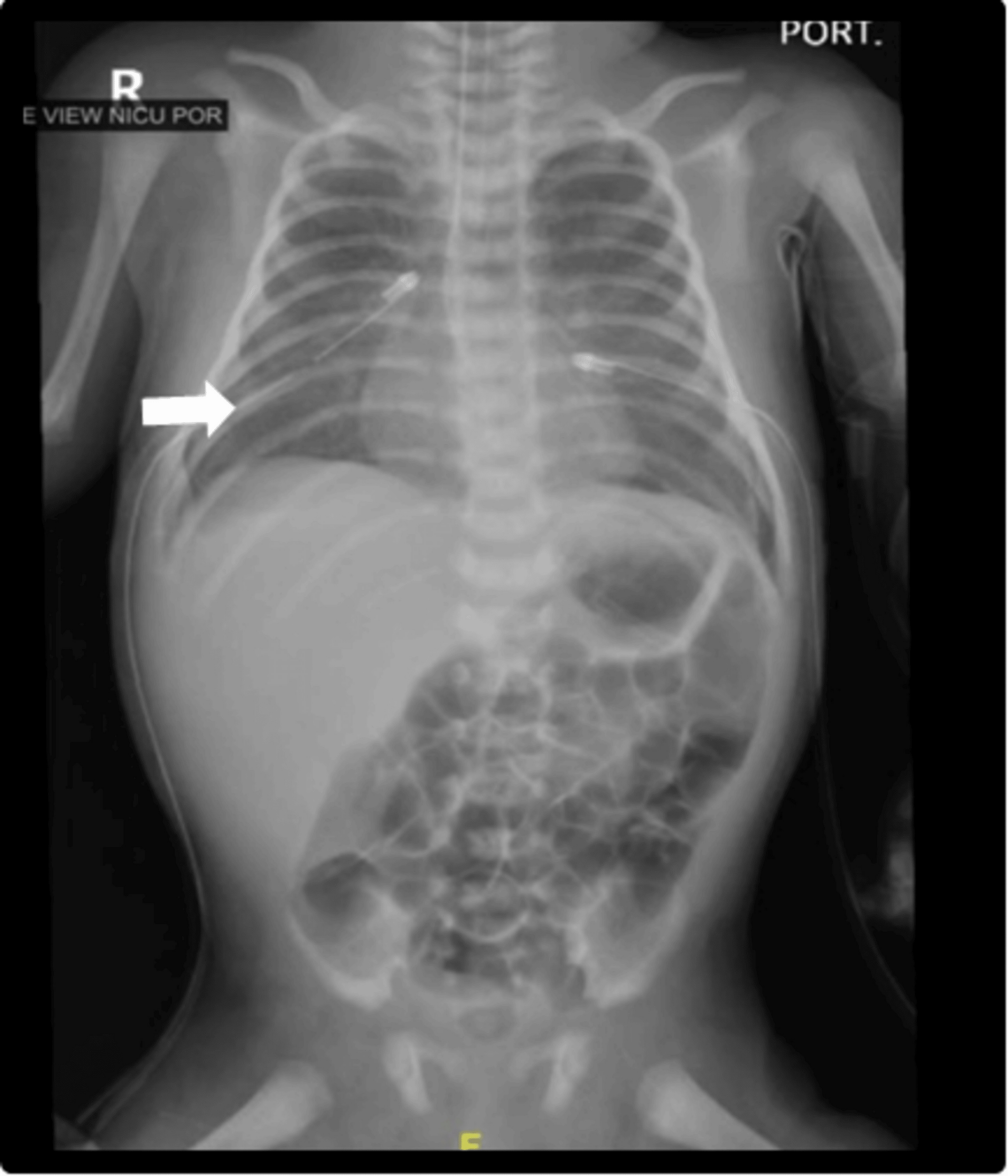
Chest and abdominal X-ray of a neonate with congenital nephrotic syndrome with bilateral pleural effusion that improved upon insertion of chest tubes (white arrow).
